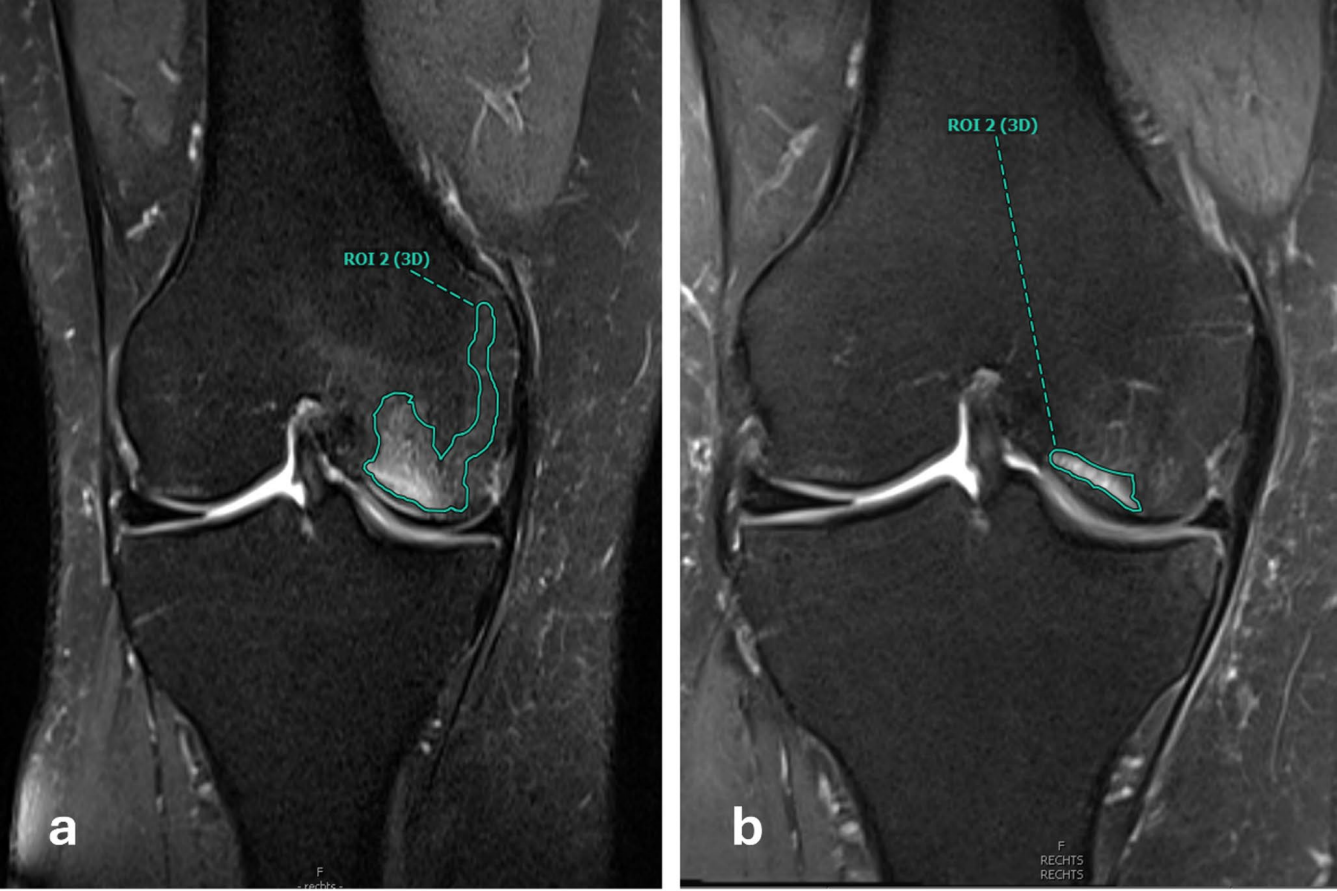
(a) of region of interest (ROI) of observer 1 in pre-treatment MRI (PD fs, coronal slicing, 3 mm thickness), (b) ROI of observer 1 in 3 months post treatment MRI (PD fs, coronal slicing, 3 mm thickness)
